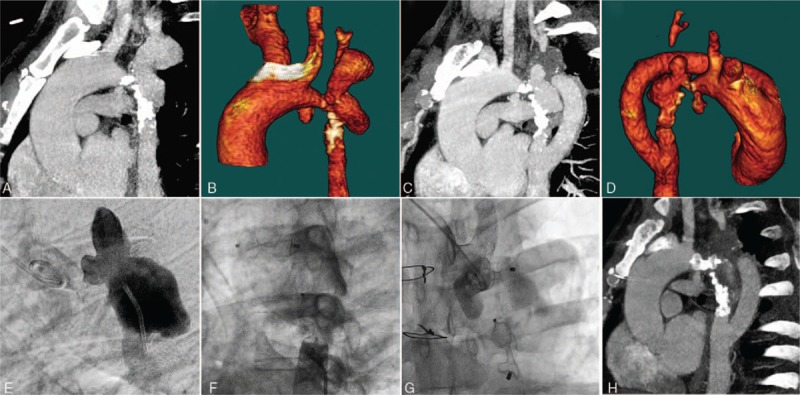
(A and B) Preoperative CTA shows an aortic arch coarctation located between left common carotid artery and left subclavian artery with a poststenotic biloculated calcified aneurysm. (C and D) Postoperative CTA shows patency of the extra-anatomic bypass graft and the aneurysm. (E) Angiography in the aneurysm shows the diameter of the proximal and distal tract connecting to the aneurysm was measured at 5 and 7 mm. (F and G) Angiography after occluders deployment distal to the aneurysm and proximal to the aneurysm shows that the aneurysm was completely isolated from the aortic cavity. (H) CTA at 3-month follow-up confirmed the absence of contrast leakage into the aneurysm and patency of the bypass graft. CTA = computed tomography angiography.
